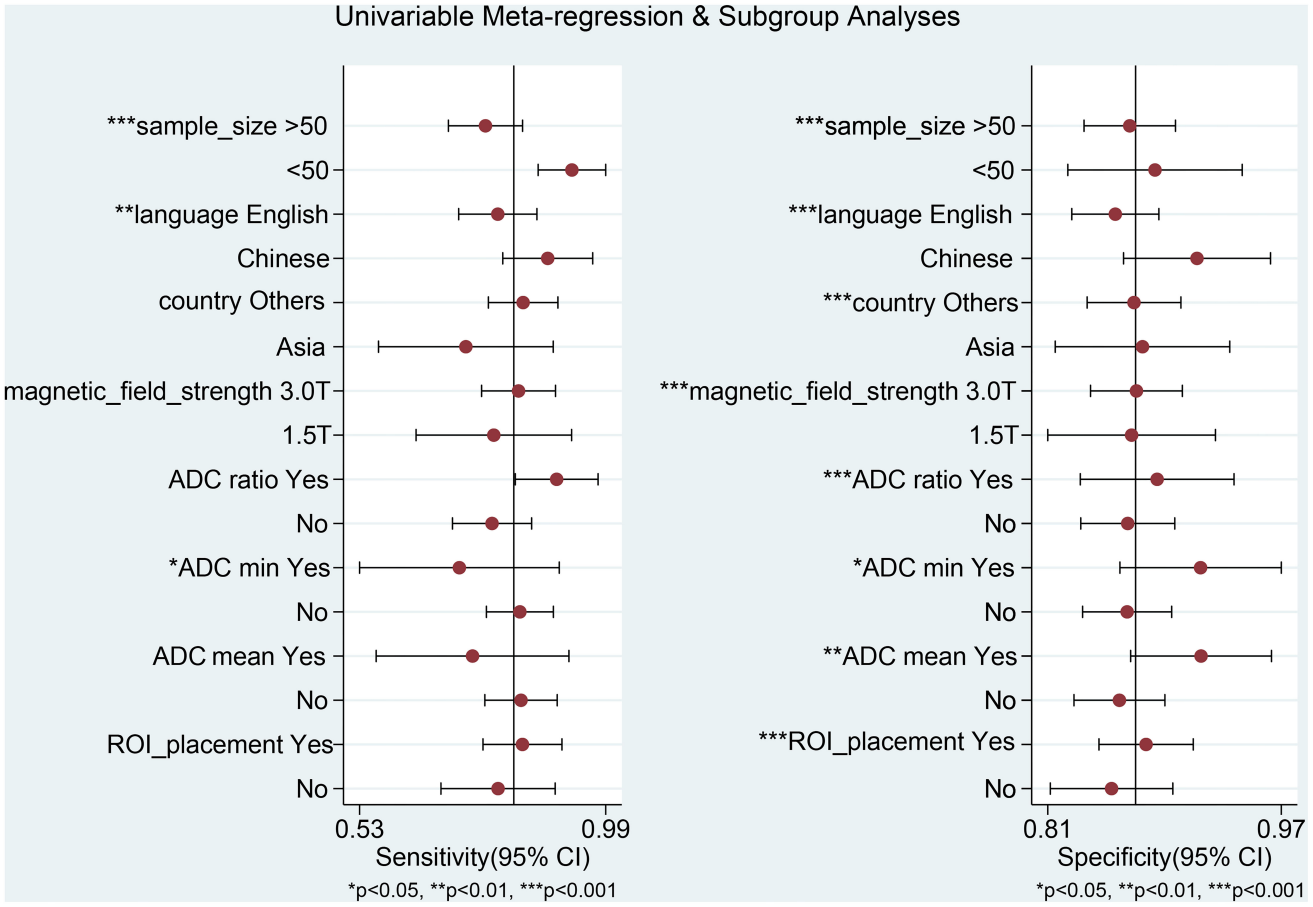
Univariable meta-regression analysis for the sensitivity and specificity of DWI for differentiating between primary central nervous system lymphoma (PCNSL) and high-grade glioma (HGG).
